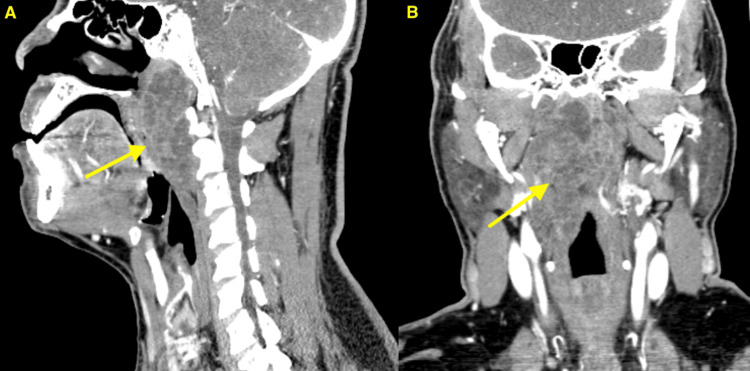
CT findings of the mass. (a) Sagittal view and (b) coronal view show a large heterogeneously enhancing soft tissue mass (yellow arrows) extending from the central and right aspect of the nasopharynx with contiguous inferior extension into the oropharyngeal soft tissues terminating just inferior to the right palatine tonsillar pillar.
